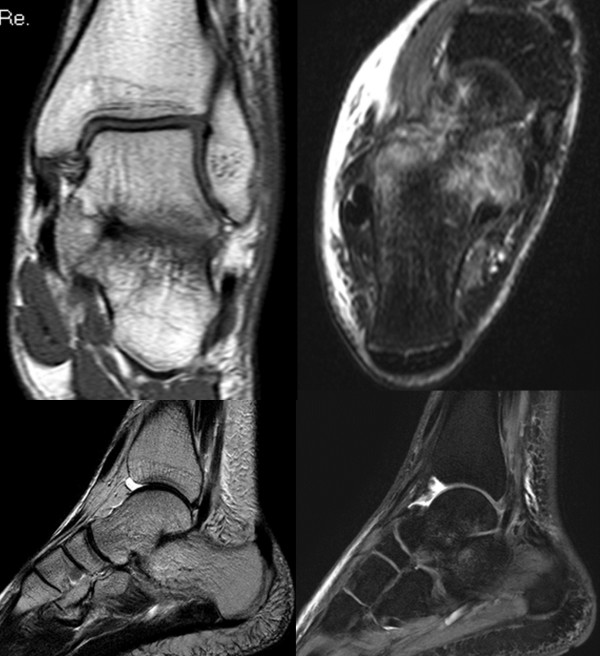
Several MRI slices showing the lateral ligament injury (left upper), the bone bruise in the calcaneus (right upper) and the coalition in the lower pictures.
